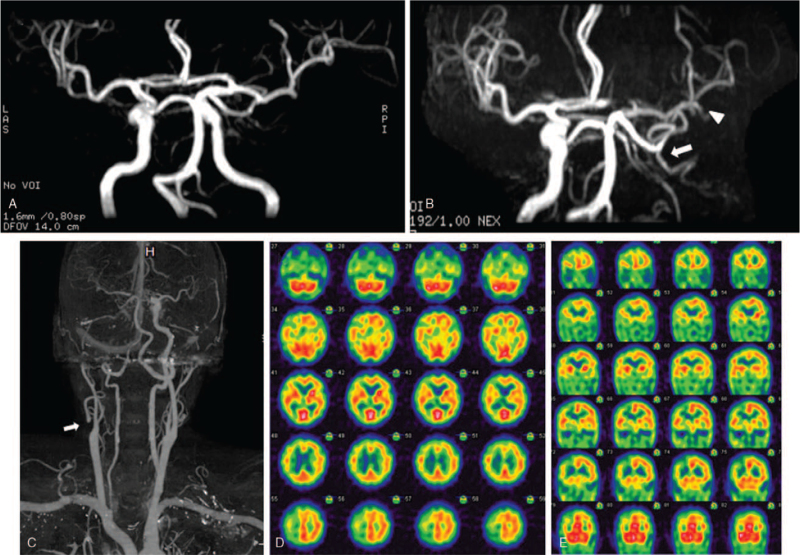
(A) Magnetic resonance angiography (MRA) taken 4 months earlier revealed patent bilateral internal carotid artery (ICA). (B) At the time of this admission, MRA revealed occlusion of the right internal carotid artery (ICA) (arrow) with resultant decreased flow in the right middle cerebral artery (MCA) (arrowhead). (C) Brain computed tomography angiography at the time of this admission revealed total occlusion of the right ICA from the carotid bifurcation to the terminus (arrow). (D, E) Tc-99m ethyl cysteinate dimer brain single-photon emission computed tomography showed hypoperfusion involving the right cerebrum, especially in the right MCA territory.
